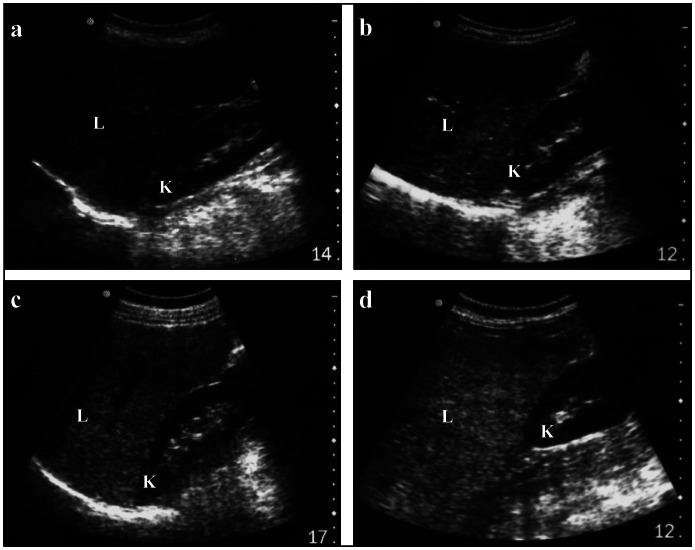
Sagittal ultrasound scans showing echo intensities in both liver parenchyma (L) and renal cortex (K). The panels represent cases in which liver steatosis was not observed (a), mild (b), moderate (c) and severe (d).
